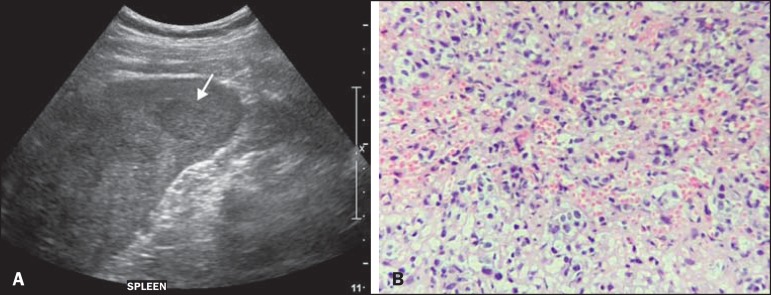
A: Ultrasound showing multiple, hypoechoic splenic nodules, one
